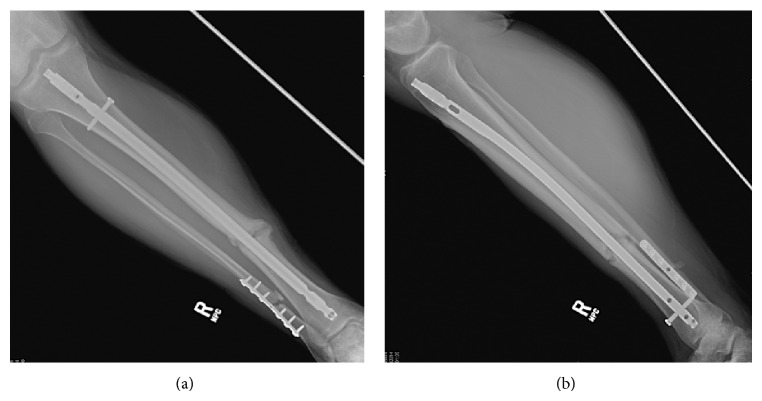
((a) and (b)) Radiographs of a hypertrophic tibial nonunion in a 35-year-old man ten months status after medullary fixation of an open tibial shaft fracture with persistent pain and inability to weight bare. Note abundant callus formation but persistent fracture line.
